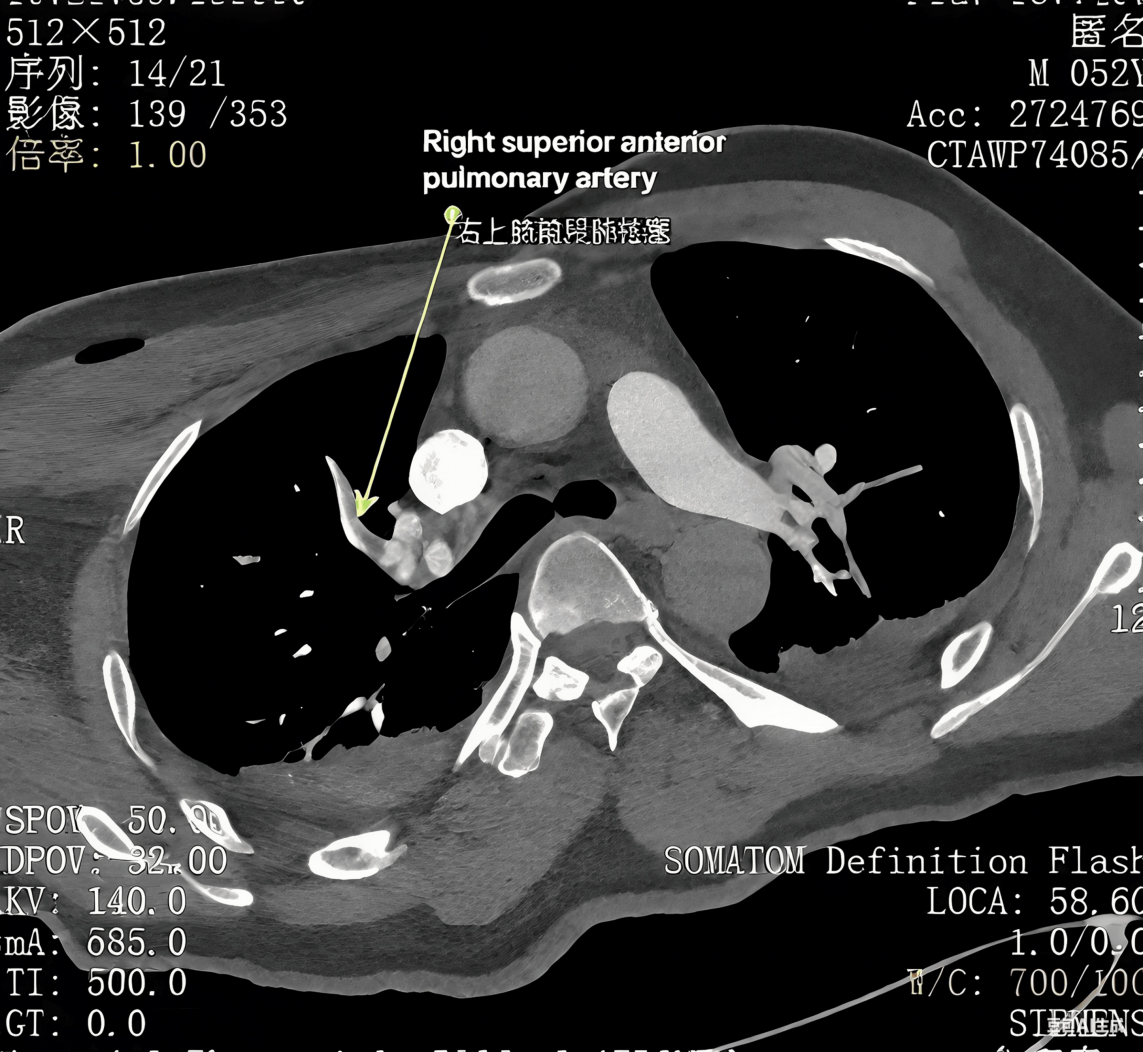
Computed tomography angiography (CTA) of the pulmonary arteries in Case 1, consistent with pulmonary embolism (PE) (arrow)
